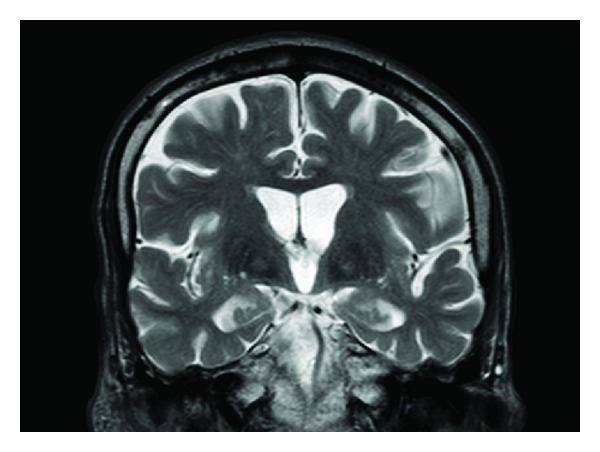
Coronal T2 weighted MRI image showing bilateral “eye of the tiger” sign in globus pallidus as well as bilateral putaminal hypointensity. While there are some areas putaminal T2 hyperintensity, they are not clearly organized into a prominent lateral hyperintense rim and adjacent discrete hypointensity as in MSA.
